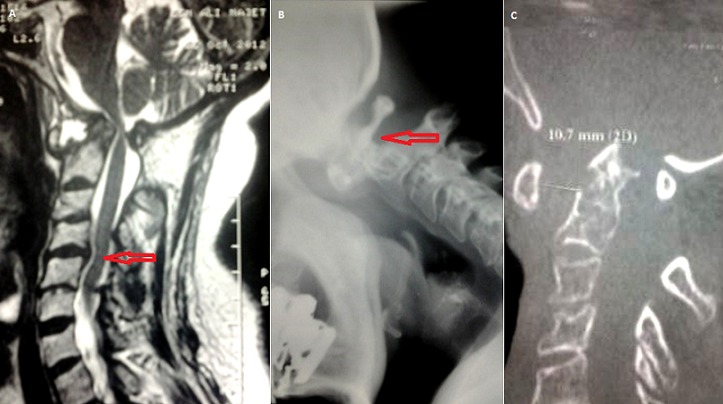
(A) IRM cervicale (coupe sagittale en T2): compression médullaire; (B) Radiographie du rachis cervical en flexion: diastasis C1-C2; (C) TDM cervicale: luxation C1-C2 et une subluxation C4-C5
